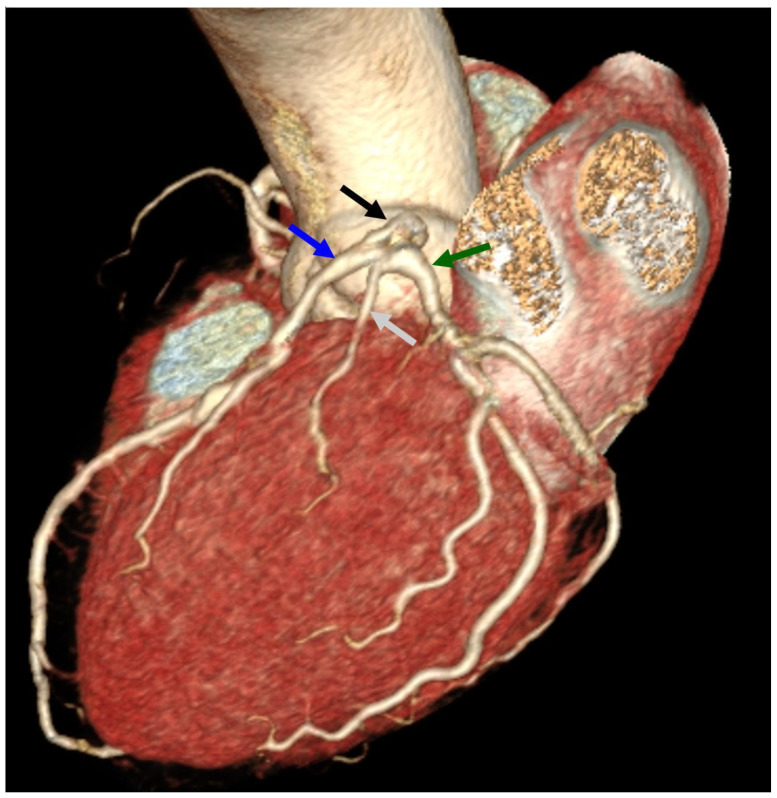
Coronary computed tomography angiography. Angiographic phase. Volume rendering reconstruction (VRT reconstruction). The black arrow indicates the left main (LM), the blue arrow indicates the left anterior descending (LAD), and the green arrow indicates the left circumflex (LCx). The gray arrow indicates the intermediate ramus (IR) resulting from the tripartite division of the LM.
